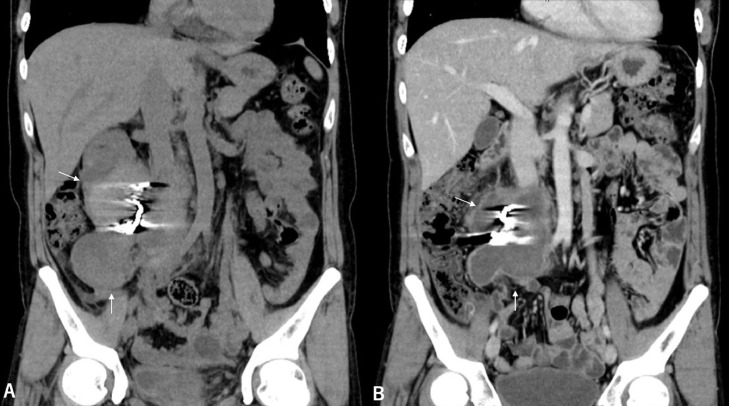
Postoperative computed tomography (11 days (A) and 38 days (B) after TAE) shows a shrinkage of the retroperitoneal hematoma. (White arrow).
